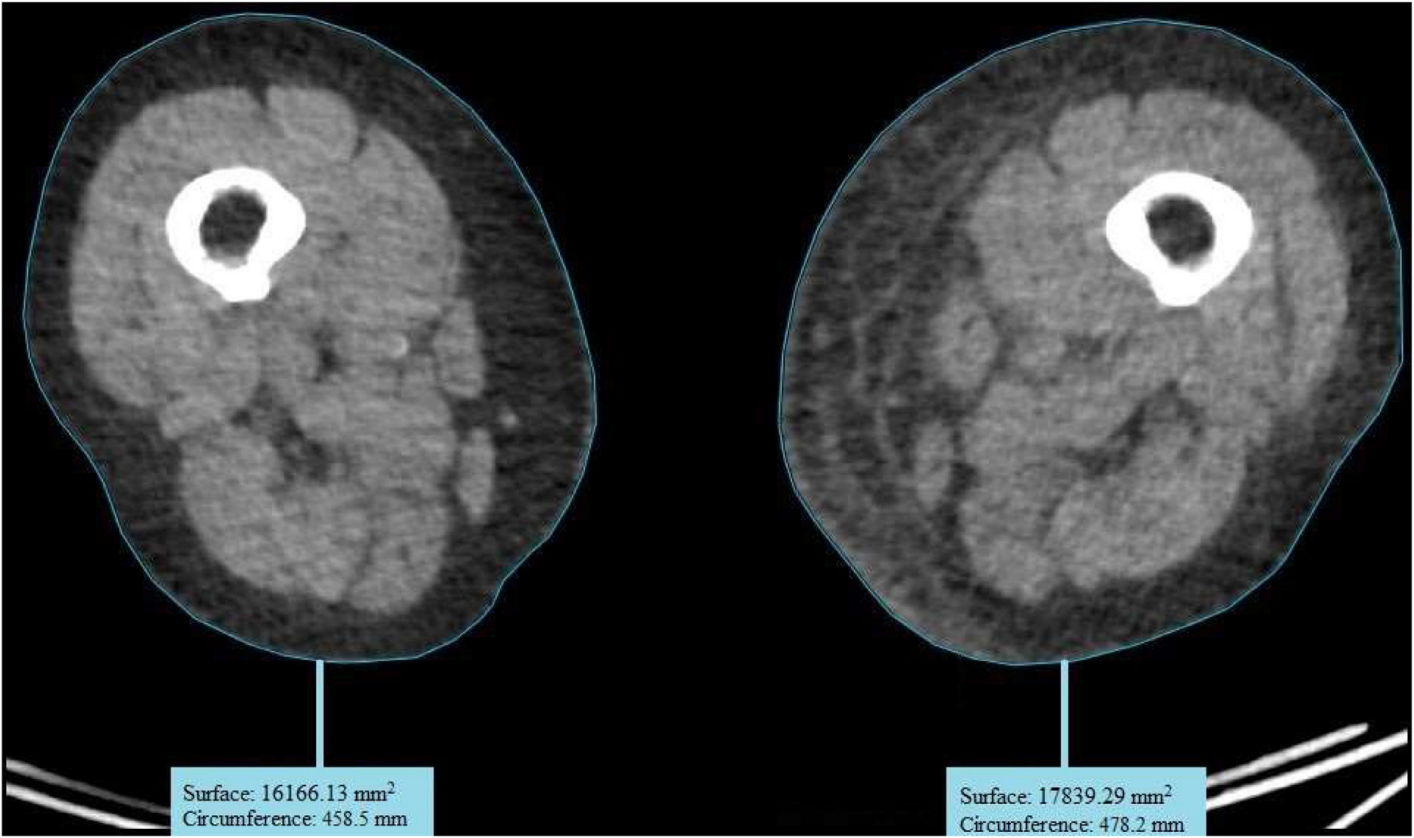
Example of thigh measurement using PET-CT
